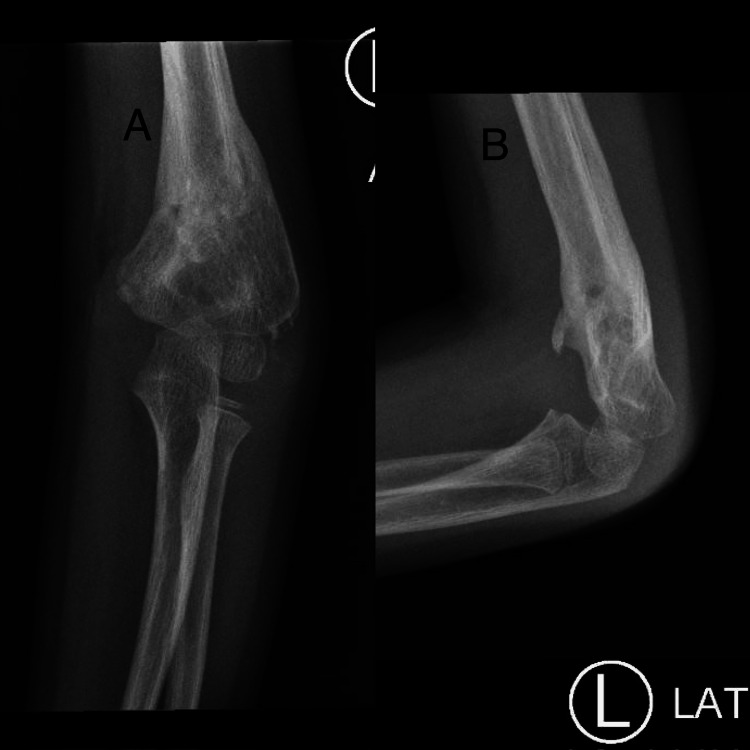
X-ray at the three-month follow-up (A) anteroposterior view; (B) lateral view
